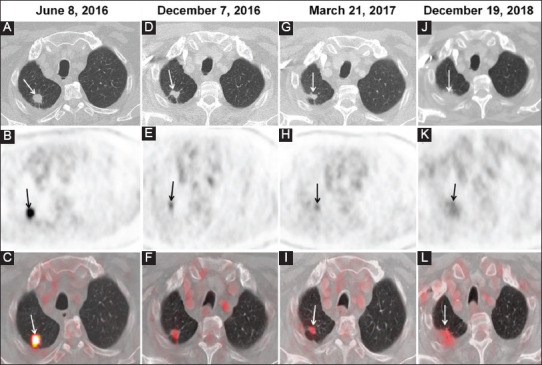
A 78-year-old male with severe COPD (chronic obstructive pulmonary disease) and domiciliary oxygen therapy. Incidental finding of lung nodule on chest X-ray. 18F-fluorodeoxyglucose (^18^F-FDG) positron emission tomography/computed tomography (PET/CT) images before stereotactic body radiation therapy (SBRT) (A, B, and C) show a solid spiculated nodule with FDG uptake and SUVmax 13,7 suspicious for primary lung cancer (arrows). The patient was treated with SBRT in October 2016. ^18^F-FDG PET/CT images in December 2016 (D, E, and F) and March 2017 (G, H, and I) showed reduction in size and lower FDG uptake (SUVmax 4.1 and 3.8, respectively) (arrows). ^18^F-FDG PET/CT in 2018 showed a subpleural atelectasis with SUVmax 3.6 (J, K, and L) (arrows).
